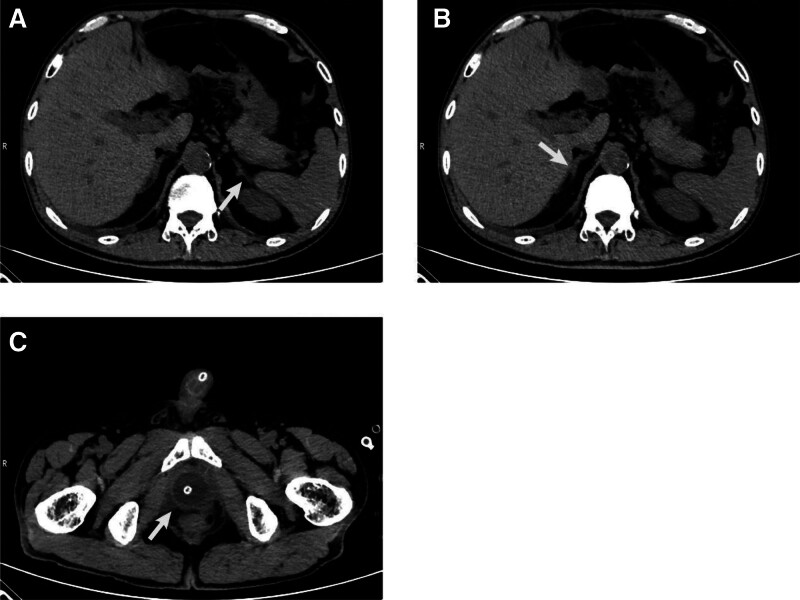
CT of the patient 1 month after surgery. No significant abnormalities were found in the left (A) and right (B) adrenal glands. (C) No significant abnormalities were observed after radical cystectomy.
